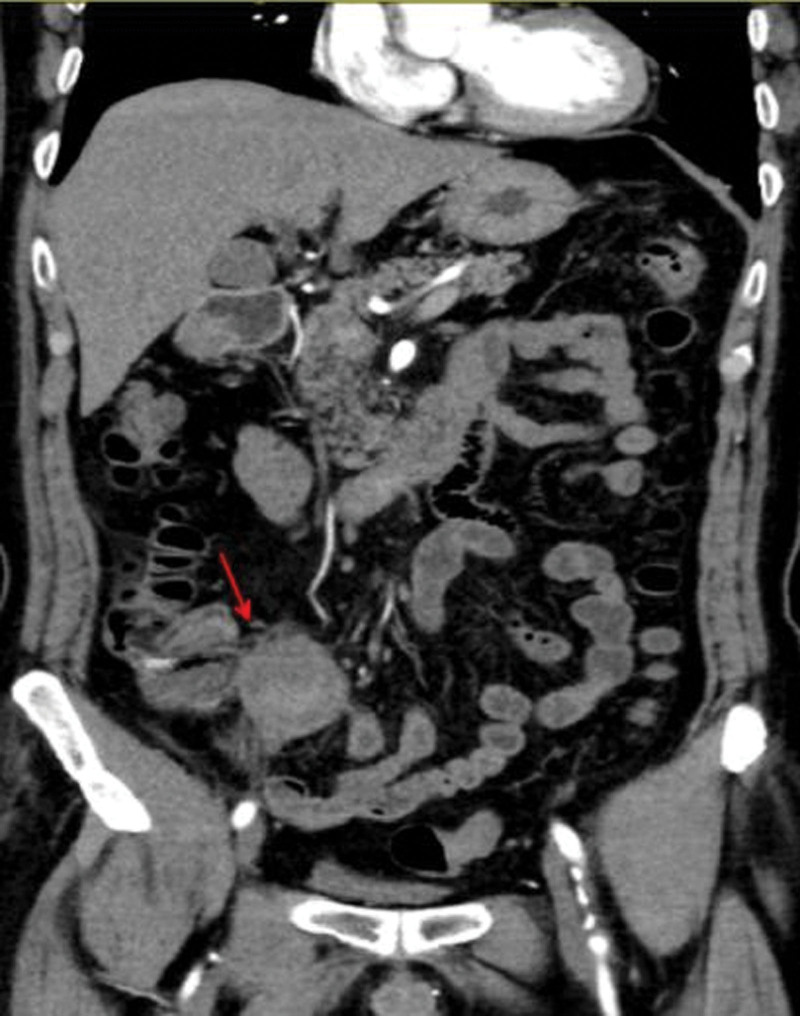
Abdominal CTA examination showing obvious intestinal edema with intestinal adhesion in the ileocecal region. CTA = computed tomography angiography.
